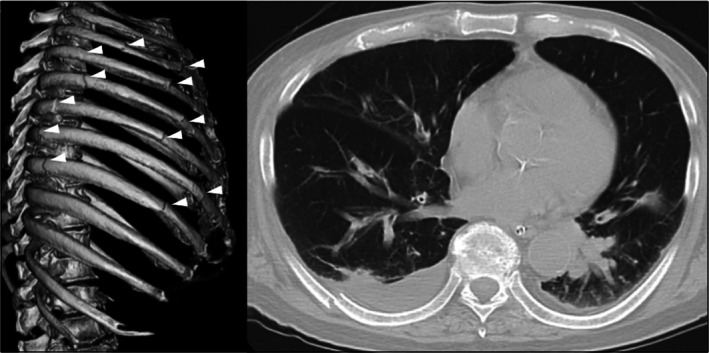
Chest computed tomography images showing multiple rib fractures (arrowhead) and areas of atelectasis.
